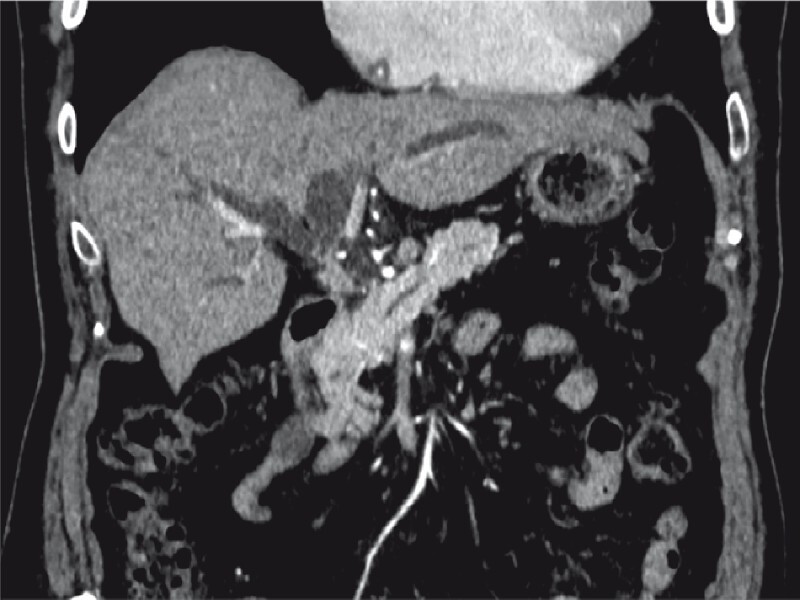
Contrast-enhanced computed tomography scan showing a Bismuth–Corlette type II perihilar cholangiocarcinoma.
